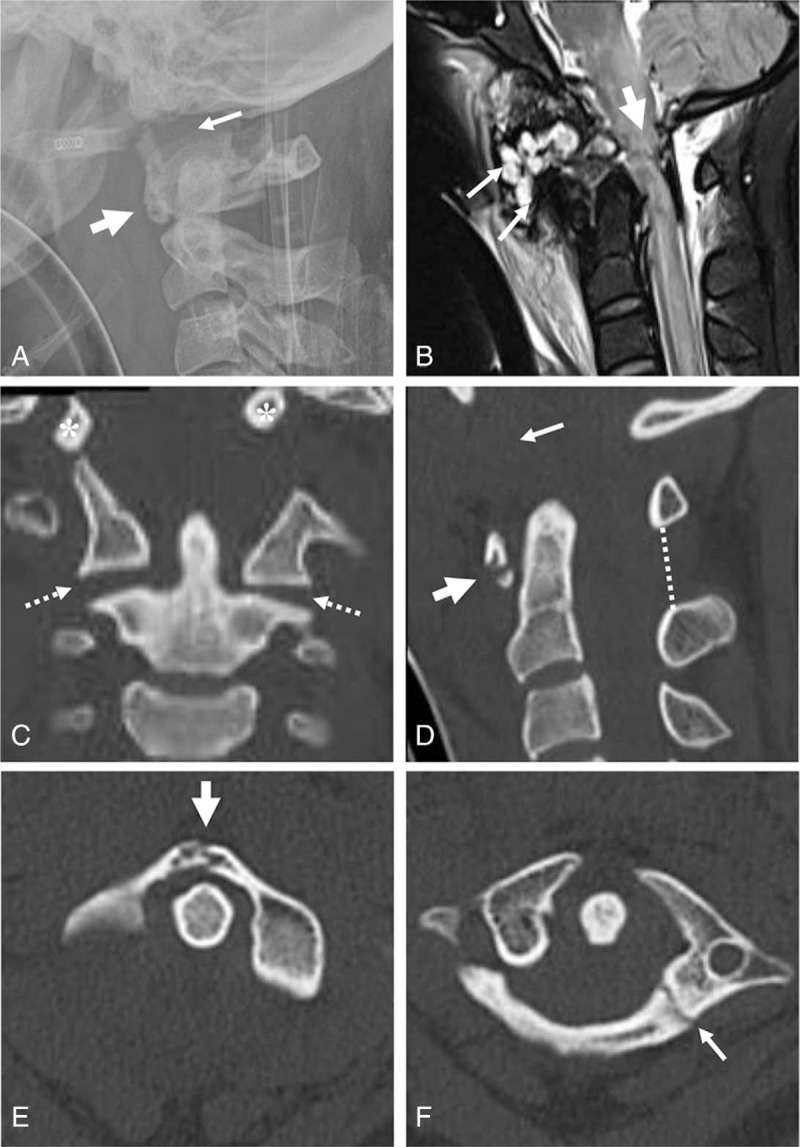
Lateral radiograph of the cervical spine revealed increased soft tissue swelling, horizontal split fracture of the anterior arch of the atlas (thick arrow), and increased basion-dental interval of 24 mm (arrow) (A). Sagittal MRI revealed complete rupture of the ligamentous complex (white arrows) and severe intramedullary cord hemorrhage at the occipito-cervical junction (thick arrow) (B). Coronal 2-D CT revealed vertical displacement of the occipital condyles (asterisks), and increased atlanto-axial joints (dotted arrows) (C). Sagittal 2-D CT revealed horizontal split fracture of the anterior arch of the atlas (thick arrows), increased atlanto-axial joints (dotted arrows), and increased basion-dental interval of 24 mm (arrow) (D). Axial CT revealed 2-part fracture of the atlas, including anterior arch fracture (thick arrow) and posterior arch fracture (arrow) (E and F). Parasagittal 2-D CT scans demonstrated vertical displacement of the occipital condyles (asterisks) with respect to the lateral mass of the atlas and posterior arch fracture of the atlas (arrowhead) (G and H). At 1 year post-surgery, plain radiographs of the cervical spine revealed solid fusion of the occipitocervical junction (I and J).
